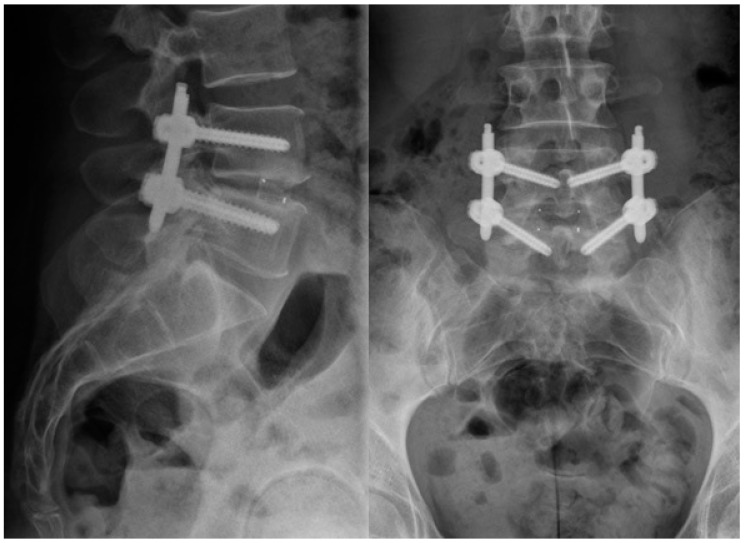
anterior–posterior (right) and lateral (left) X-ray of an 40 year old woman after TLIF surgery with percutaneous dorsal instrumentation and implantation of the newly-developed cage at the level L4/5, one-year FU.
